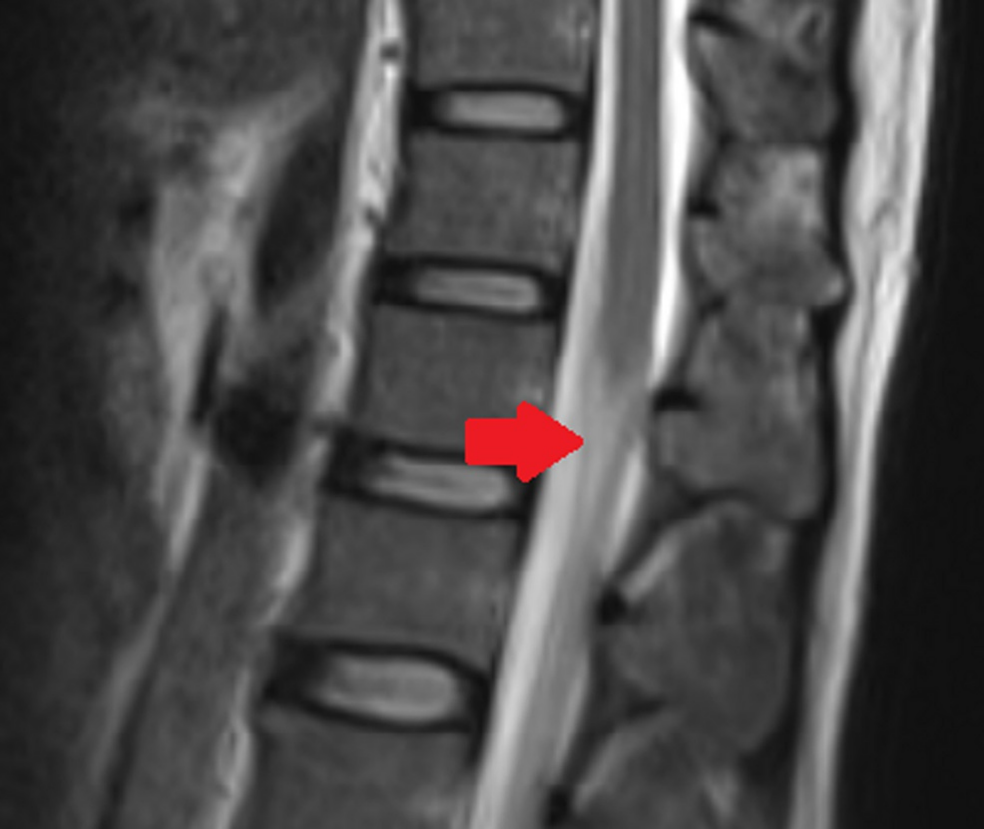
MRI spine showing surface enhancement over the conus medullaris.
